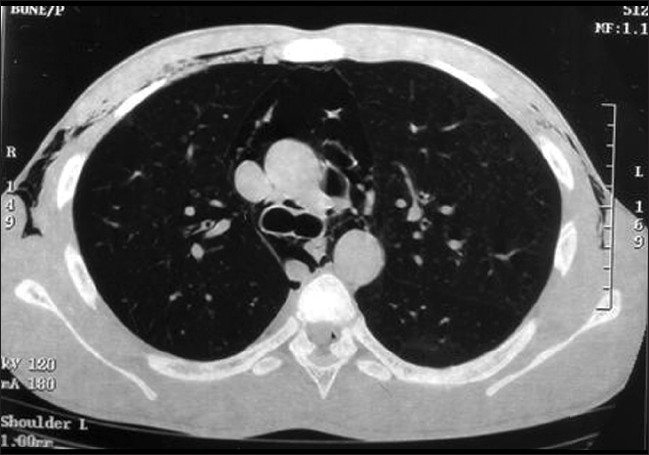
CT chest showing pneumomediastinum, pneumopericardium and surgical emphysema
